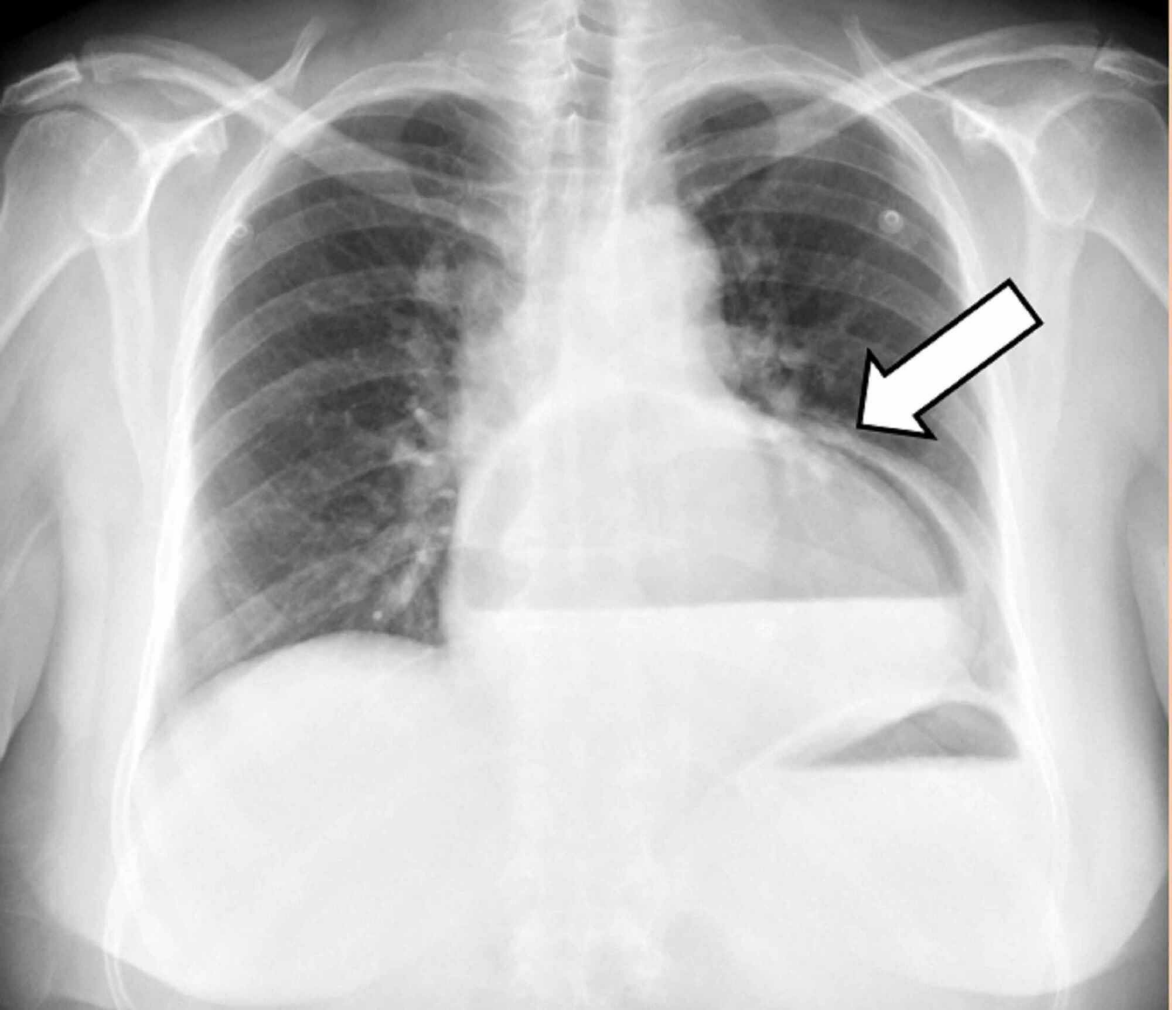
Chest radiograph showing a retrocardiac air-filled mass (white arrow)
